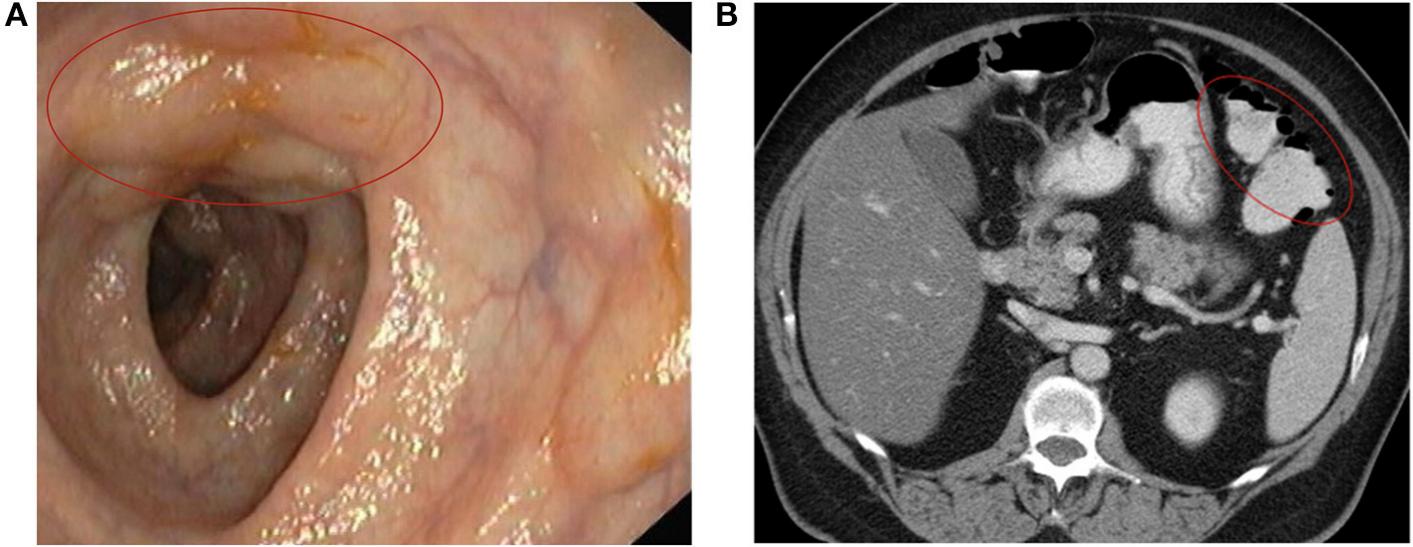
Pretreatment images of a 59-year-old woman with PCI that underwent HBOT at our centre (69) (A) PCI's endoscopic image of subepithelial nodular elevations covered with mucosa of normal appearance (B) Abdominal computed tomography showing gas-filled cysts in the splenic flexure of the colon.
